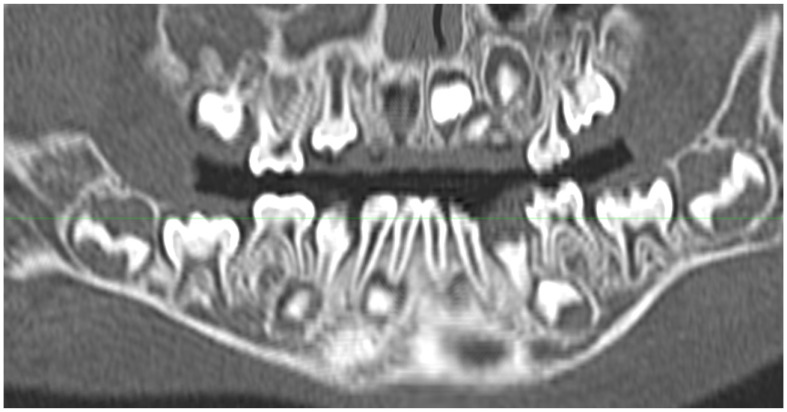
Curved plane coronal CT reconstruction in a 28-month-old child, presented in a manner similar to that of panoramic tomography. The horizontal green line reflects an image processing artifact.
